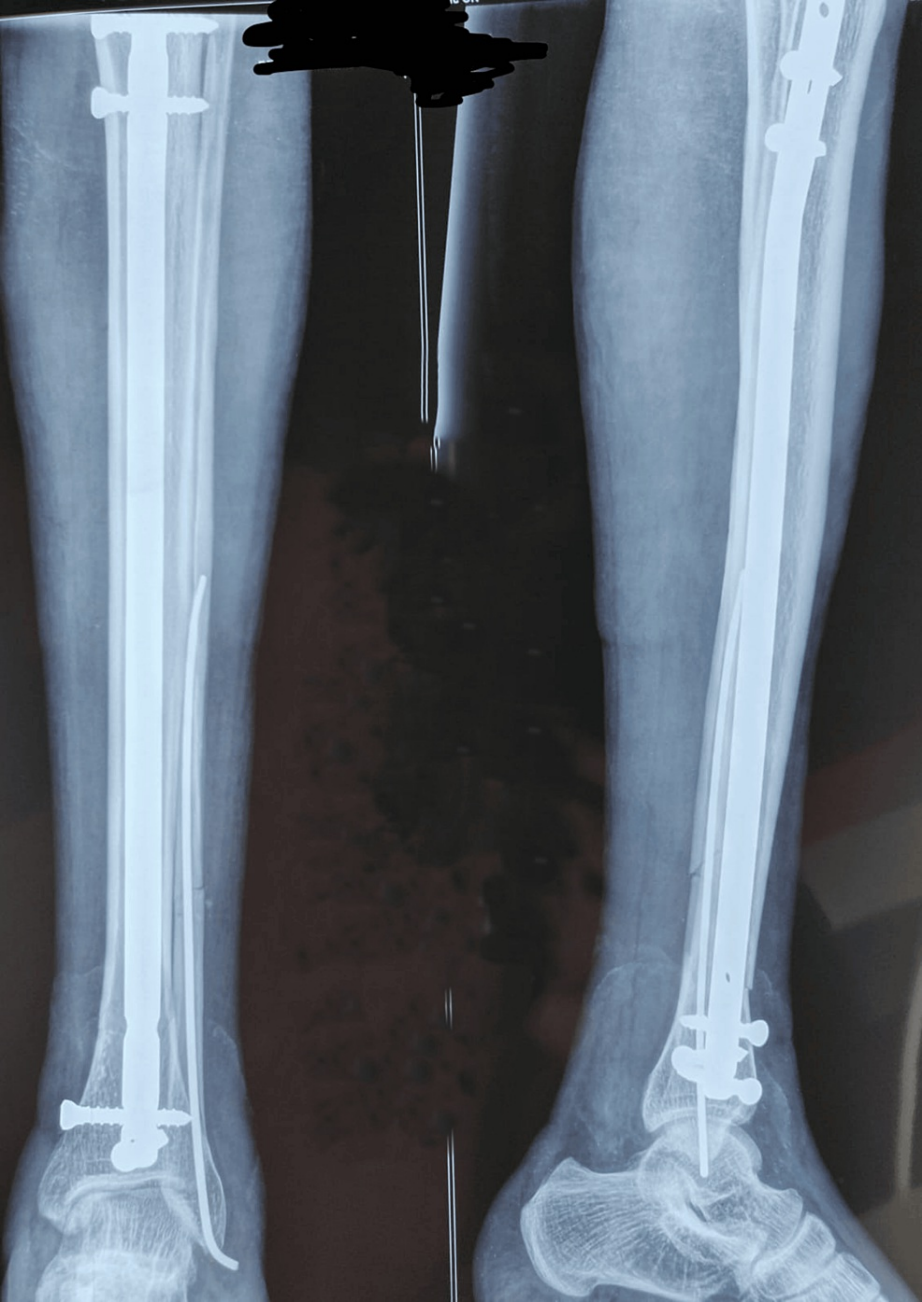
Post-operative radiograph
